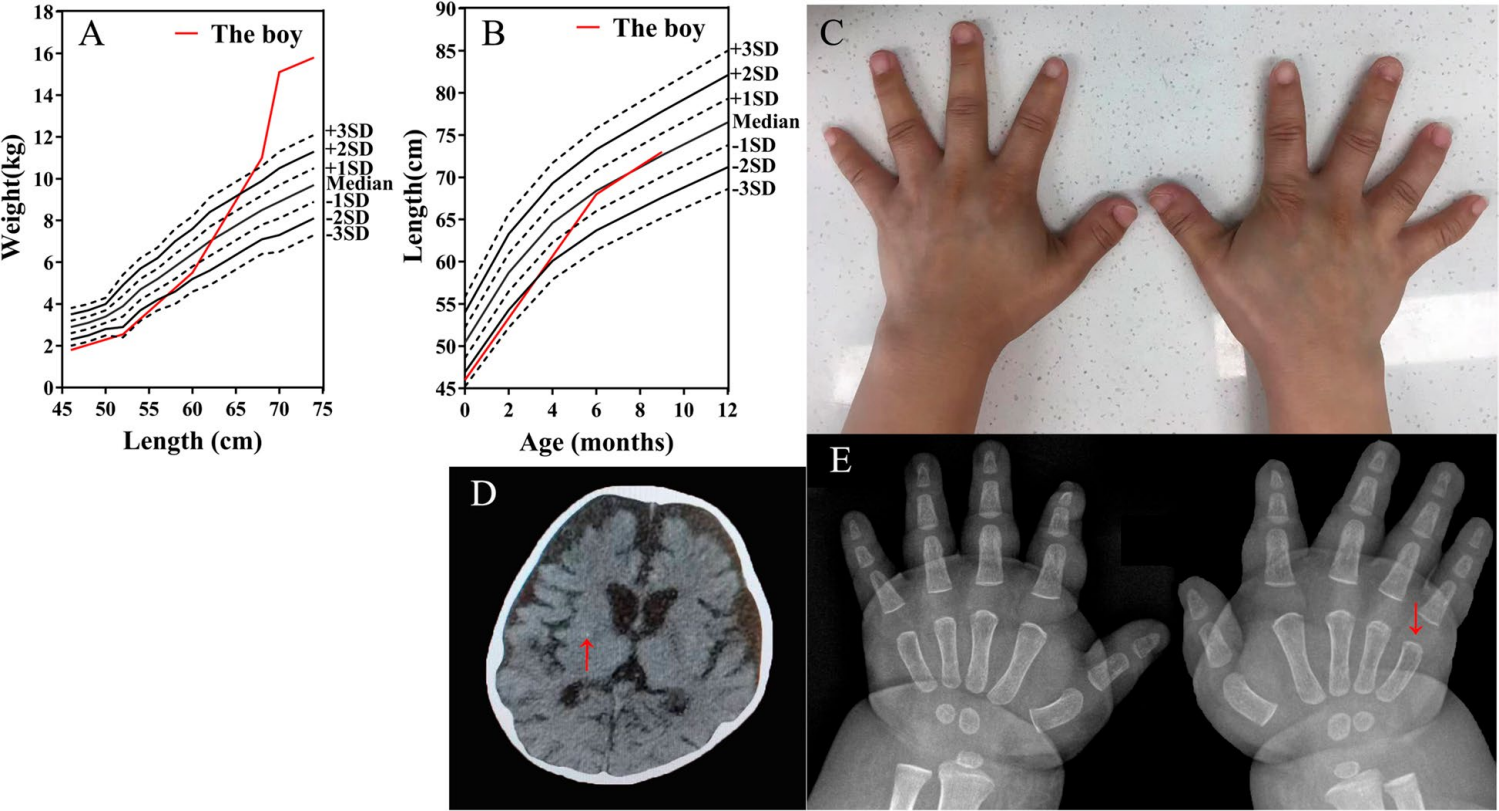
Clinical phenotype of the proband and his mother. A Weight-for-length Z-score curve of the proband according to the standards for children in China. The red color represents the proband’s accelerated weight gain. B Length-for-age Z-score curve of the proband according to the standards for children in China. C Brachydactyly from the proband’s mother. D Cranial computed tomography of the proband demonstrated right focal basal ganglia calcification (arrow). E Radiograph of the hands demonstrated the proband’s shortened 5th metacarpal on the right hand (arrow)
